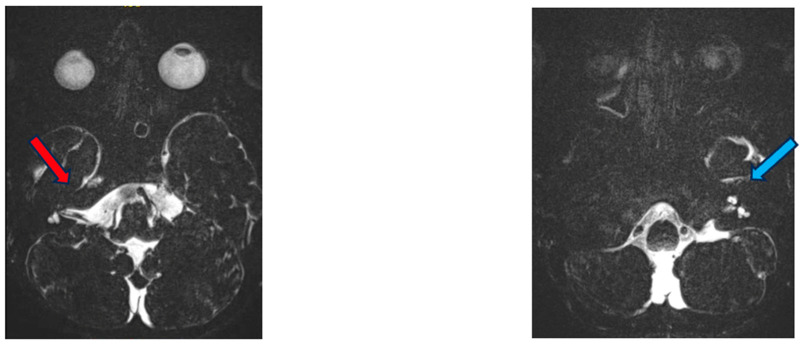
MRI of the inner ear for case 1, T2 tse3d weighted image, dysplasia of the lateral and vestibulum bilaterally (blue arrow depicts dysplastic canal on the left, red on the right).
